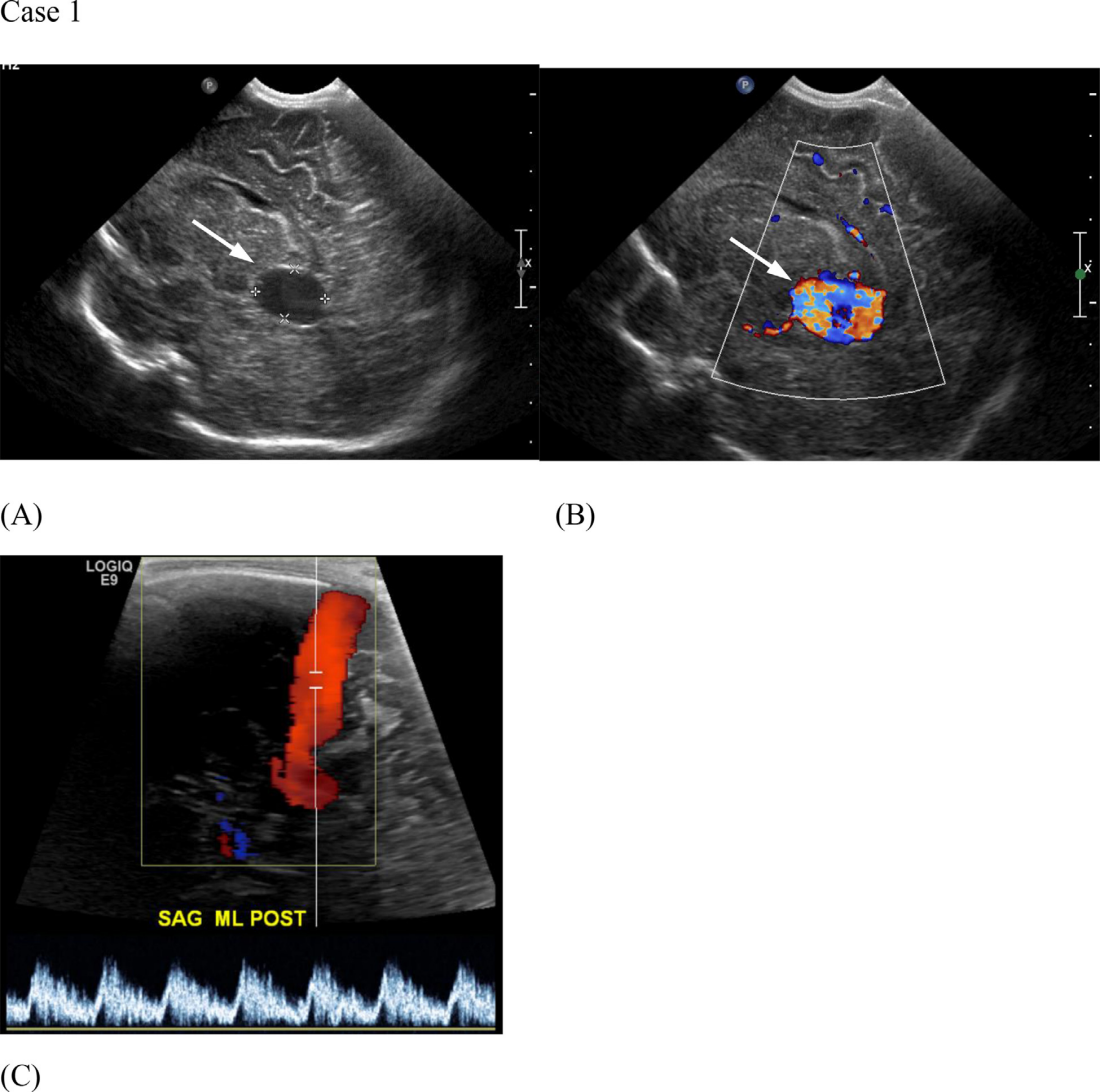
(Case 1) Head US without (A) and with (B) color Doppler demonstrate a vein of Galen malformation (arrows). Spectral Doppler (C) demonstrates a persistent falcine sinus extending superiorly from vein of Galen malformation.
